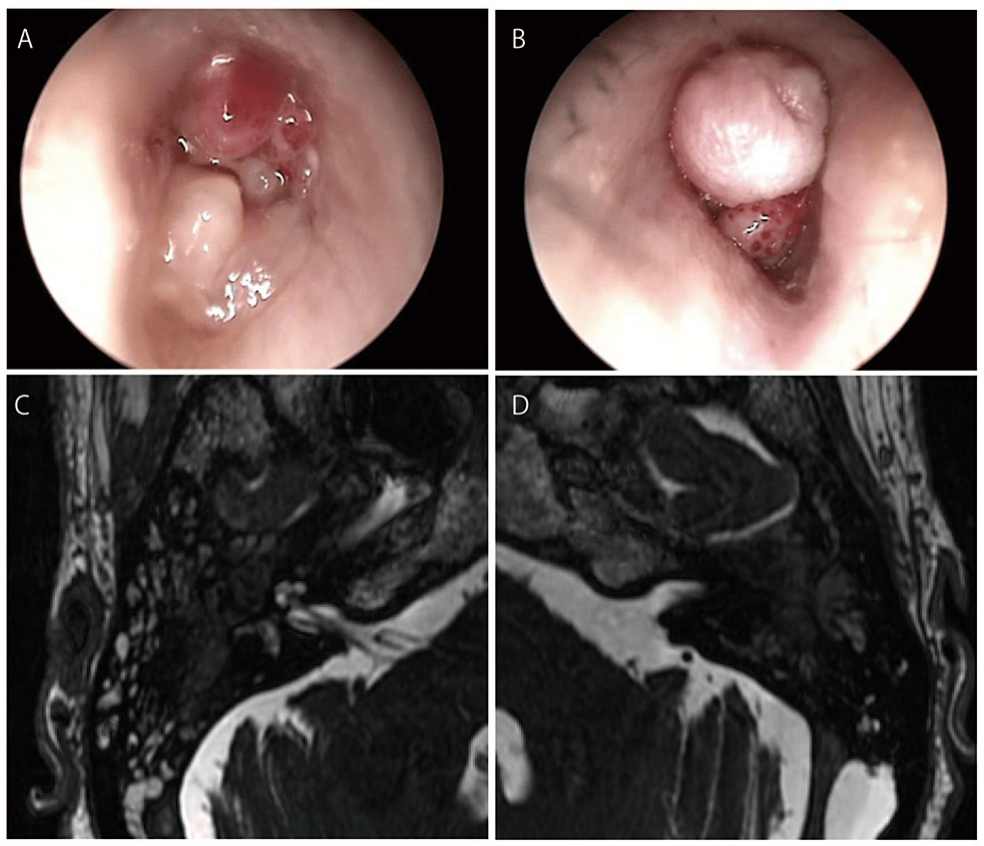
Physical examination findings before cochlear implantation (A, B) Cochlear implantation of the tympanic membrane. Increased granulation filled the ear canal compared with the initial examination. (C, D) Preoperative magnetic resonance imaging. The left cochlea is filled with soft tissue. In contrast, residual fluid is present in the right cochlea.
